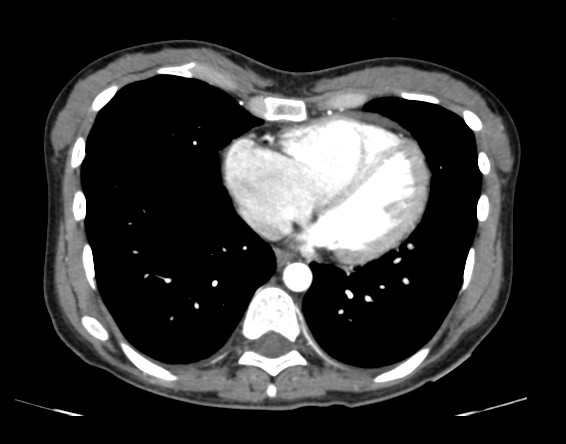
CT axial series with medastinal window after infusion of intravenous contrast, showing increase in the size of the right atrium and right ventricle. The right ventricular to left ventricular ratio is approximately 1.8.
